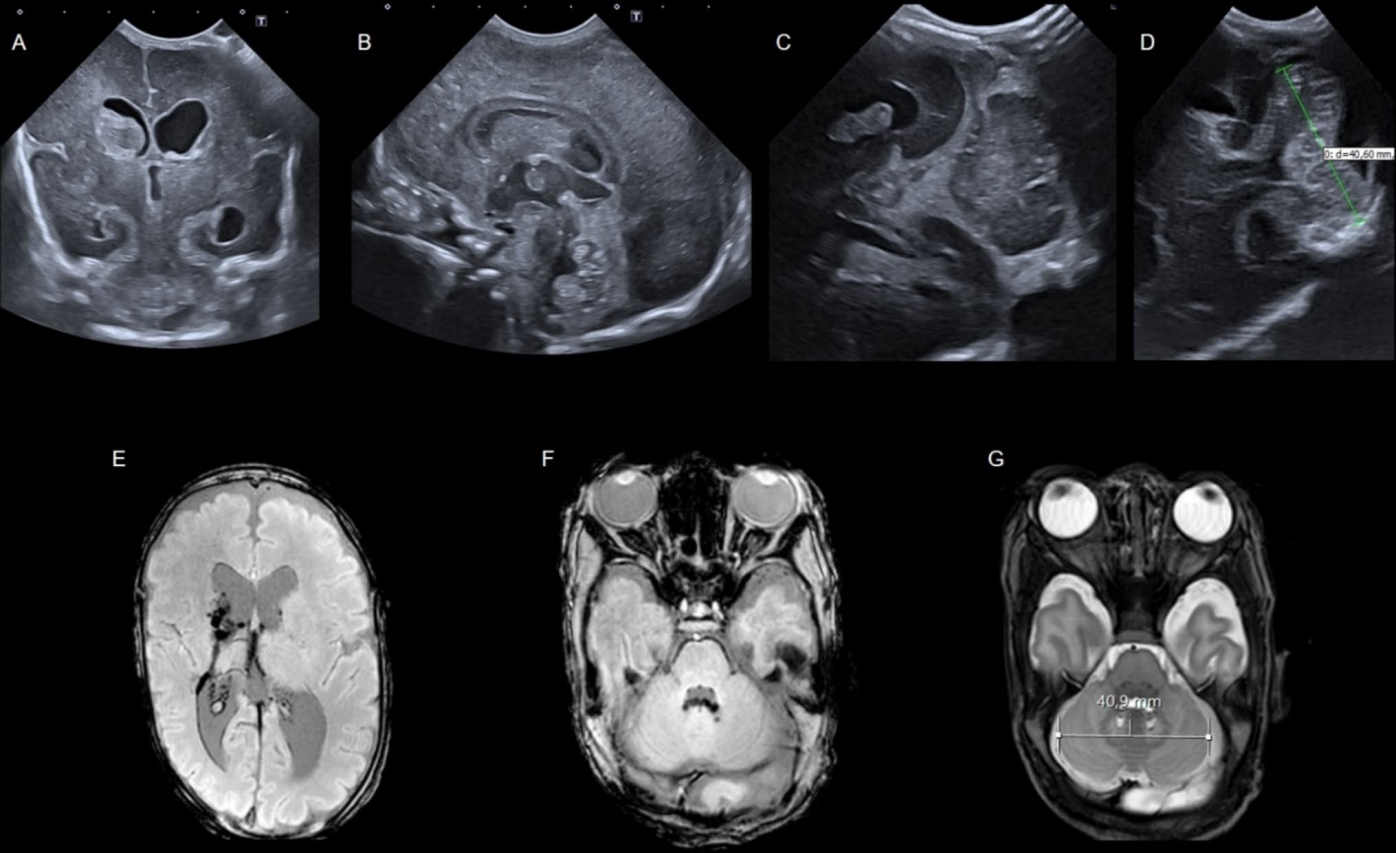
Impaired cerebellar development after high-grade intraventricular hemorrhage in a preterm infant of 25 weeks gestational age. A Coronal scan shows IVH III° right side with posthemorrhagic ventricular dilatation. B Sagittal midline scan shows an enlarged III^rd^ ventricle and prominent IV^th^ ventricle and blood clots within the posterior fossa around the cerebellar vermis and in the cisterna magna. C coronal scan through the mastoid fontanel shows even more pronounced the infratentorial blood clots in the cerebrospinal fluid around the otherwise unaffected cerebellum. D coronal scan through the mastoid fontanel at term equivalent age with severe cerebellar hypoplasia, TCD 40.6 mm. (E + F) MRI SWI proves hemosiderin deposition mainly in right lateral ventricle and IV^th^ ventricle, especially the lateral apertures. No parenchymal hemorrhagic infarction in the cerebrum or cerebellum could be identified. G T2w scan confirms the symmetric cerebellar hypoplasia seen in US scan
